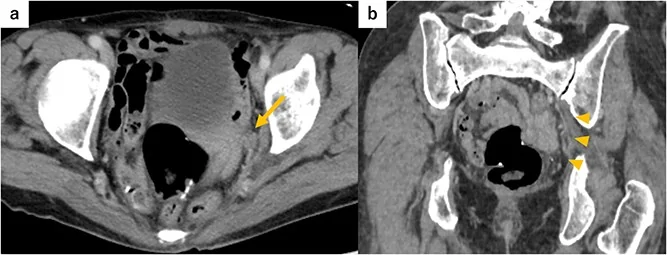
One-year postoperative CT findings without evidence of recurrent ureterosciatic hernia; (a) axial contrast-enhanced CT image obtained 1 year postoperatively showing no evidence of recurrent herniation of the ureter into the sciatic foramen (arrow); (b) coronal contrast-enhanced CT image obtained 1 year postoperatively demonstrating complete resolution of the sciatic hernia (arrowhead).
